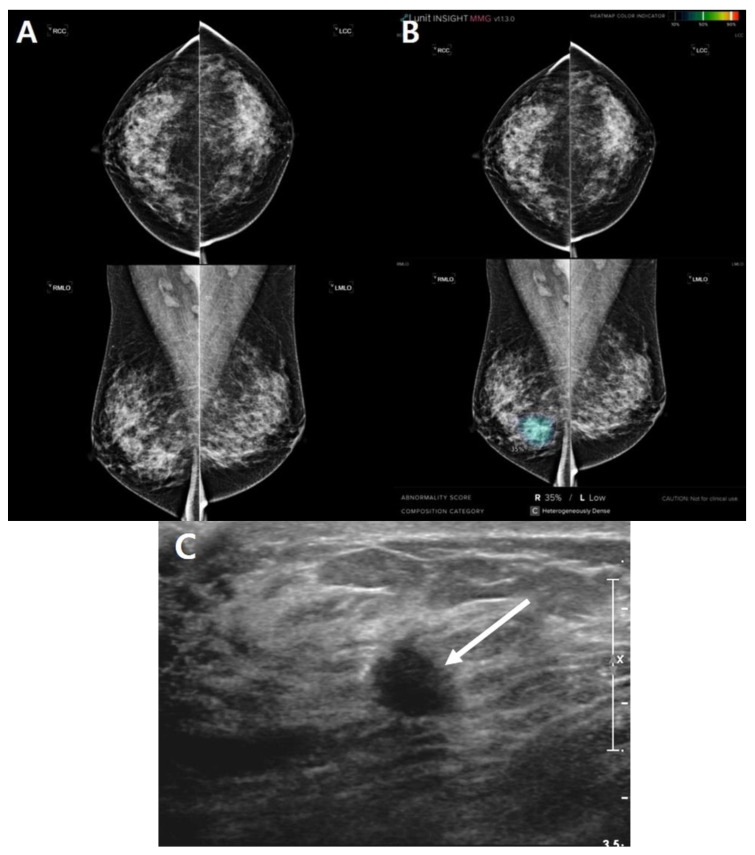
An invisible case in radiologist’s analysis that is concordant only in AI analysis. A 49-year-old woman had a breast checkup. (A) Mammography revealed a dense breast without any abnormal lesions in both breasts. (B) However, artificial intelligence highlighted a suspicious area on the right mediolateral oblique view and presents an abnormality score of 35%. (C) Ultrasound revealed a 0.8 cm irregular hypoechoic mass with angular margin in the right lower central breast (arrow). Ultrasound-guided core needle biopsy revealed invasive ductal carcinoma.
